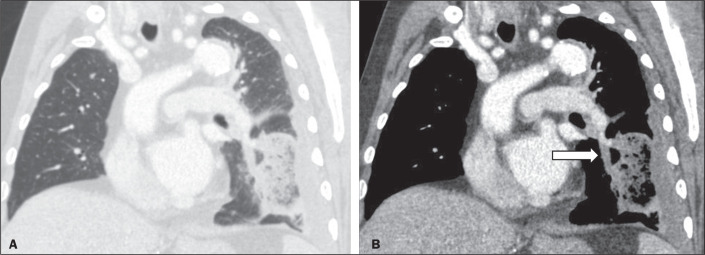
Oblique CT images showing an RHS in a lung window (A) and pulmonary
